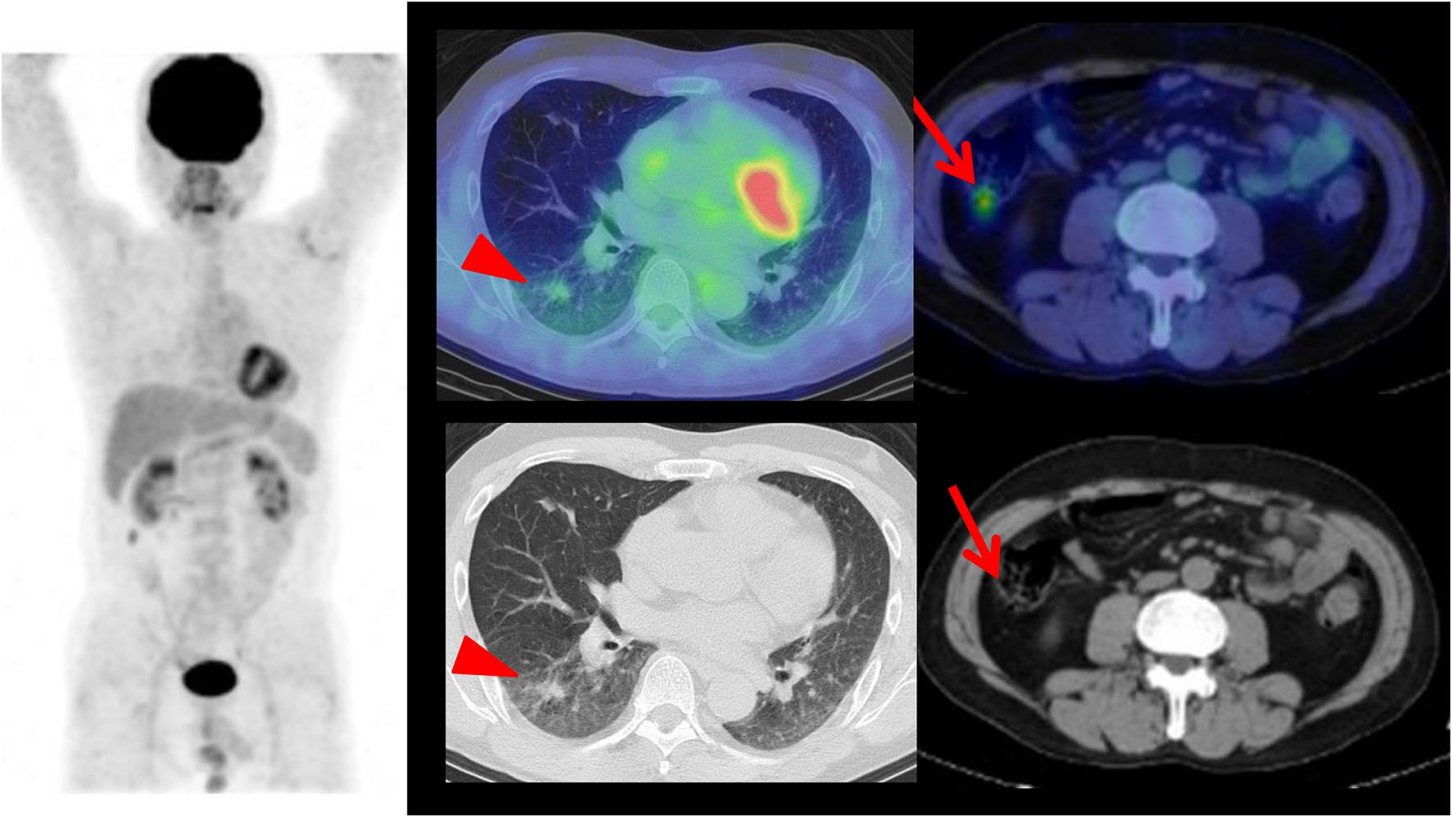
A 54-year-old male with dermatomyositis and interstitial pneumonia has been treated with corticosteroid. Whole-body FDG PET maximum projection image did not show increased FDG uptake in the systemic muscles probably due to the treatment effect. Cross-sectional FDG PET/CT images showed slightly increased FDG uptake (SUVmax 1.7) in the lung nodule of the right lower lobe (arrowhead), and focal FDG uptake (SUVmax 3.2) in the ascending colon which was diagnosed as colon cancer by endoscopic resection (arrow). Later, this patient had surgery of the right lung tumor of adenocarcinoma
